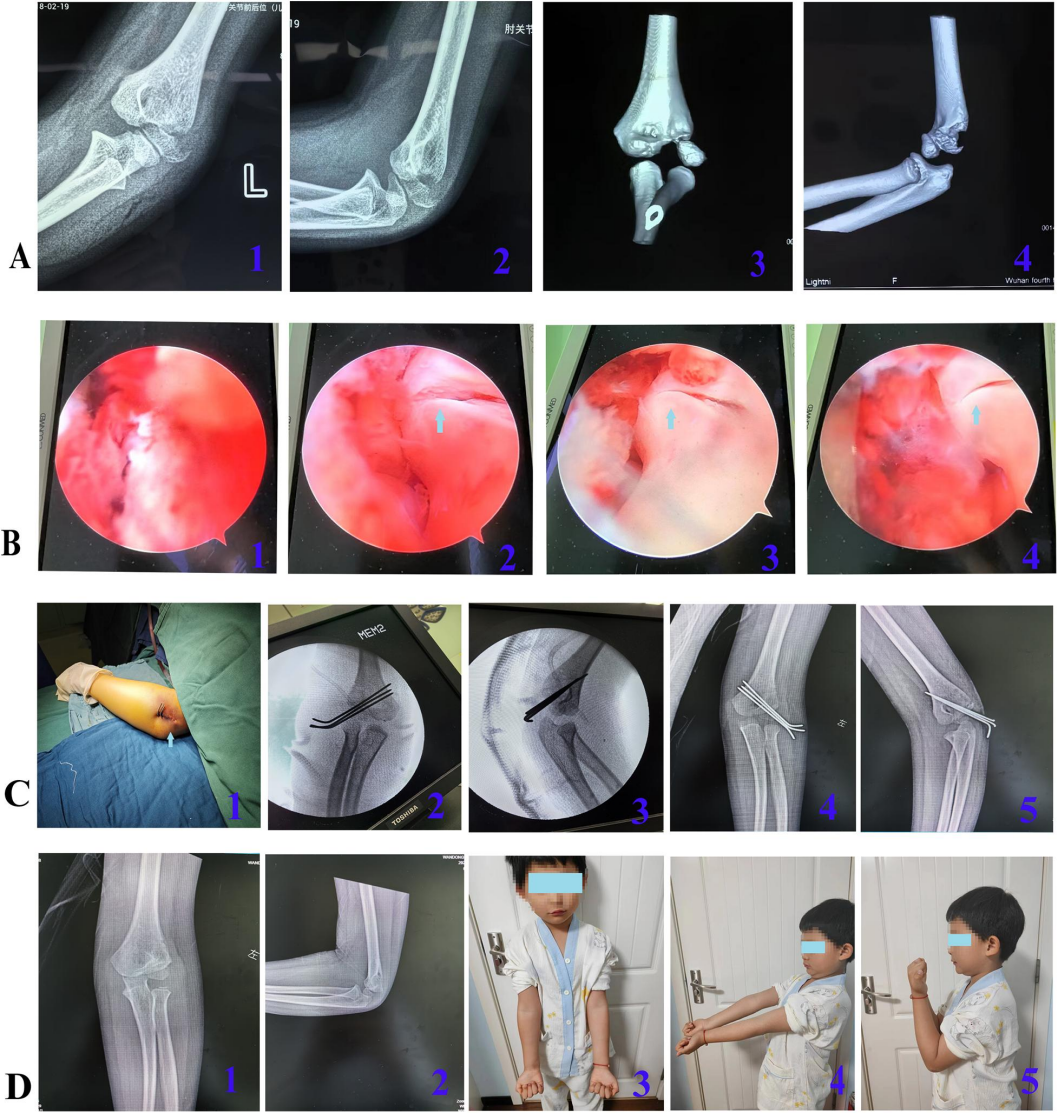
Representative case 1. A 6-year-and-7-month-old boy with a left lateral humeral condyle fracture caused by a fall. A: Preoperative imaging, including x-rays (1, 2) and CT scans (3, 4), showing a Jakob type III fracture. B: Intraoperative arthroscopic views: 1, extensive soft tissue interposition at the fracture site; 2, after debridement to relieve the interposition and preliminary reduction, converting the fracture to a Jakob type II; 3 and 4 show the post-reduction views, revealing an even articular surface and a fracture gap of <2 mm. C: 1, Postoperative appearance of the local incision; 2 and 3, intraoperative fluoroscopy images; 4 and 5, x-ray images at 1 month postoperatively. D: 1 and 2, x-ray images at 3 months postoperatively, showing fracture union; 3, 4, and 5, functional photographs at 6 months postoperatively, demonstrating no significant difference in the appearance and range of motion of the bilateral elbow joints.
